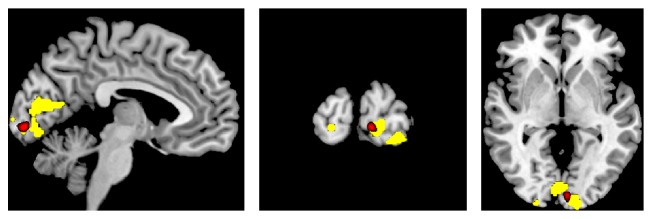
Structural alterations and functional activity following checkerboard stimulation. Functional MRI activation (yellow, MNI: *x* = −24, *y* = −94, *z* = −12; *p*_FWE_ < 0.001; *T* = 14.56) and VBM detected gray matter increase (red, MNI: *x* = −6, *y* = −93, *z* = −3; *p*_FWE_ = 0.043; *T* = 5.08) superimposed on skull striped anatomical (T1) image. GM increase and maximum BOLD signal are closely located in the primary visual cortex.
